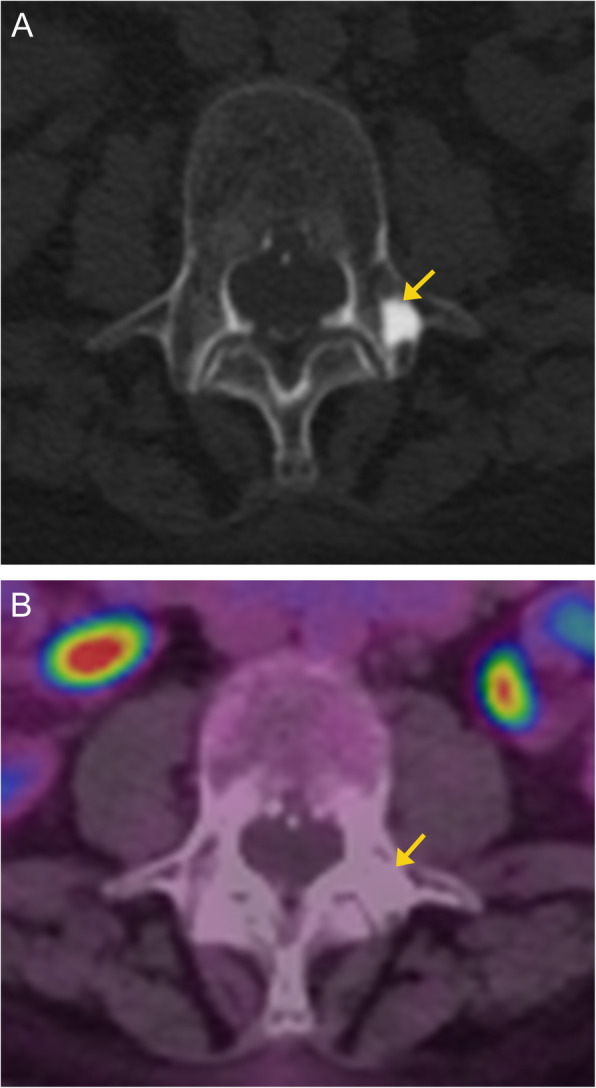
Enostosis. Axial CT image (a) of the thoracic spine demonstrates a focal sclerotic bone lesion with characteristic dense sclerosis and irregular and brush-like margins (arrow). No associated soft tissue component. Axial fused PET/CT image (b) demonstrates lack of metabolic activity of the bone lesion (arrow), confirming the diagnosis
